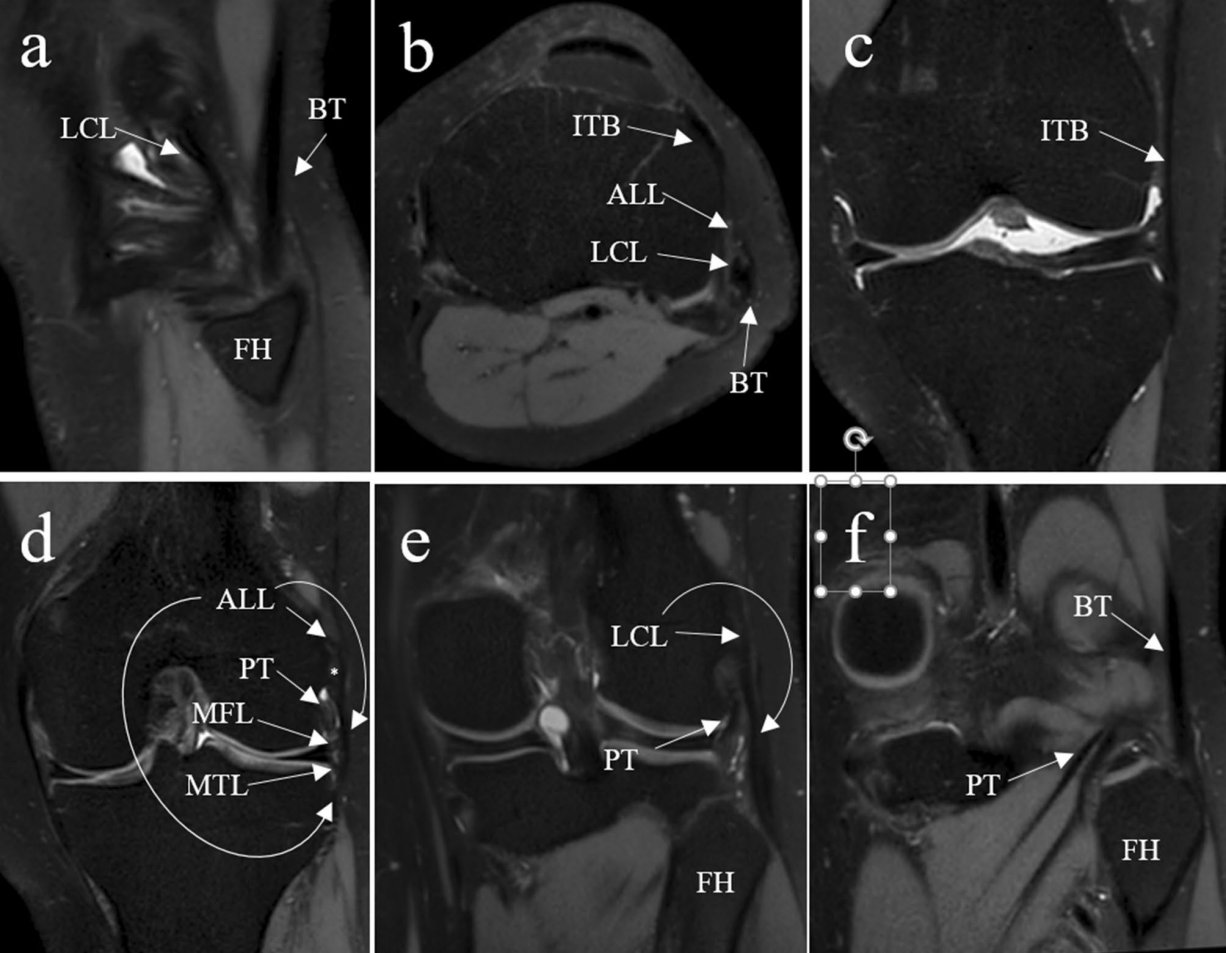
The anterolateral structures are shown in different MRI planes. (a) Represents a sagittal MRI image with the lateral collateral ligament (LCL) and the biceps tendon (BT) inserting at the fibular head (FH). (b) An axial image that displays the insertion of the iliotibial band (ITB) at Gerdy’s tubercle as well as the anterolateral ligament (ALL), LCL and BT. (c) Shows the course of the ITB in a coronal image. In image (d) the entire ALL with femoral, tibial and meniscal part is marked as well as the popliteus tendon (PT) and the meniscofemoral (MFL) and meniscotibial ligament (MTL). In this image the lateral epicondyle with the merging fibers of ALL, LCL and MFL is marked with an asterisk. Another coronal image further posterior shows the LCL and the PT (e), while in the last image (f) even further posterior the BT and PT are shown.
